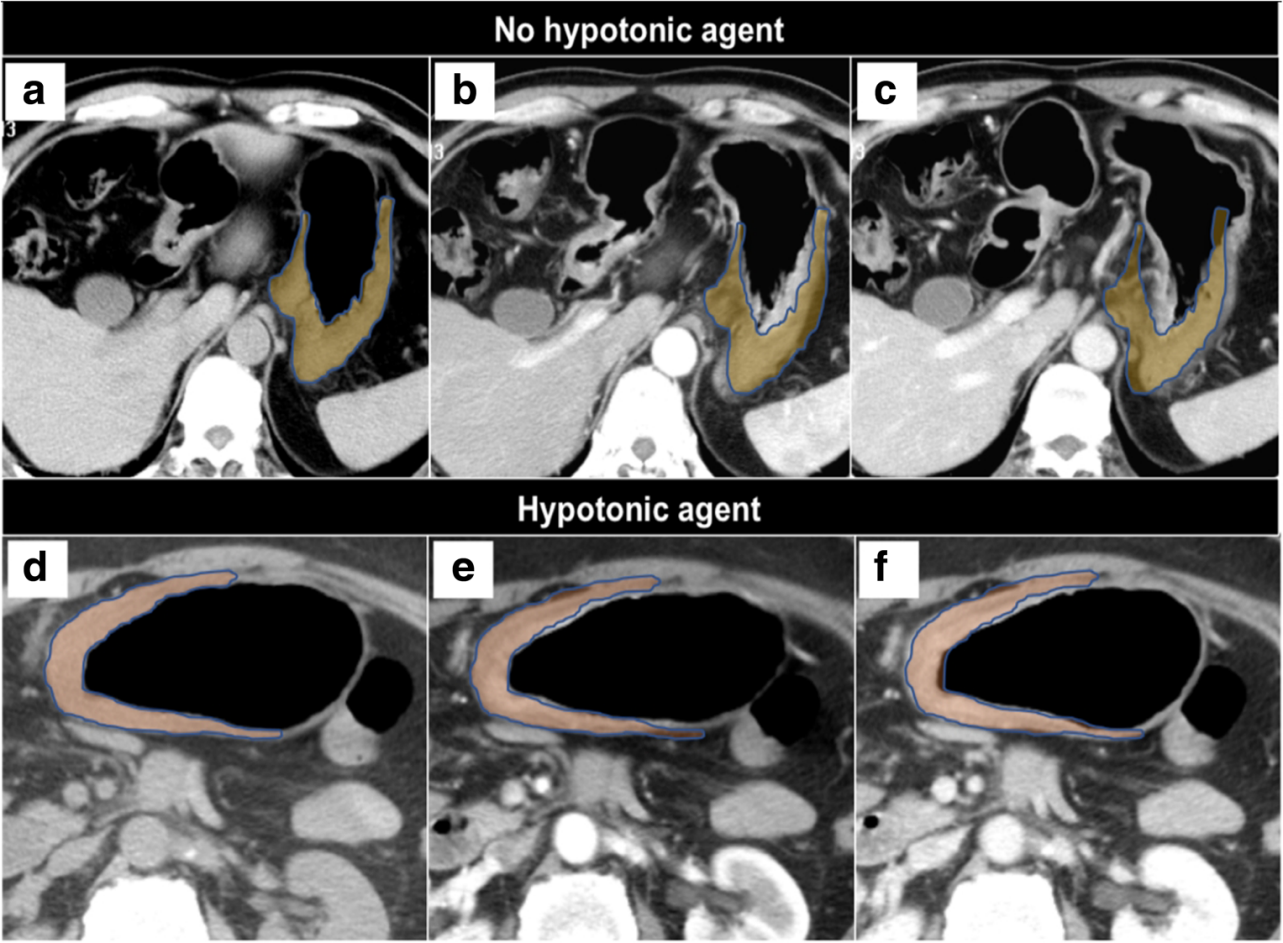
Comparison between a protocol without and with administration of anti-peristaltic agent on multidetector computed tomography. The image shows how the region of interest (ROI) delineation is more reproducible at different time points in the second scenario. Unenhanced (a and d), arterial (b and e) and portal (c and f) phases
